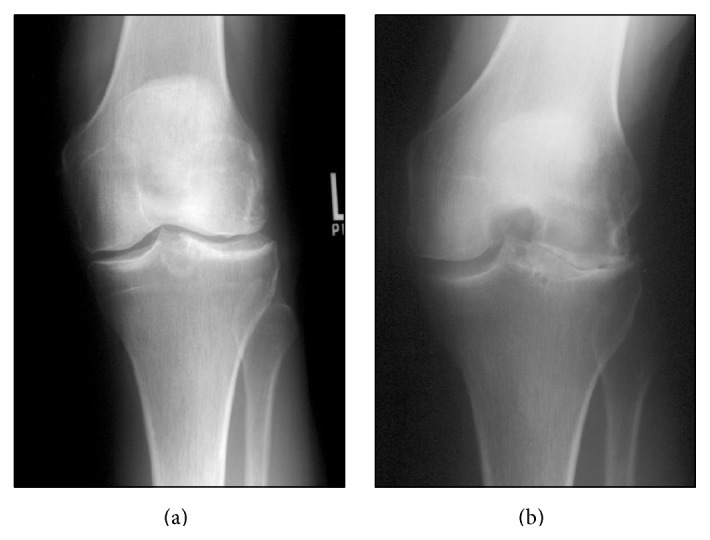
AP radiograph of a left knee (a). The tunnel view of the same knee demonstrates significant degenerative joint disease (b).
